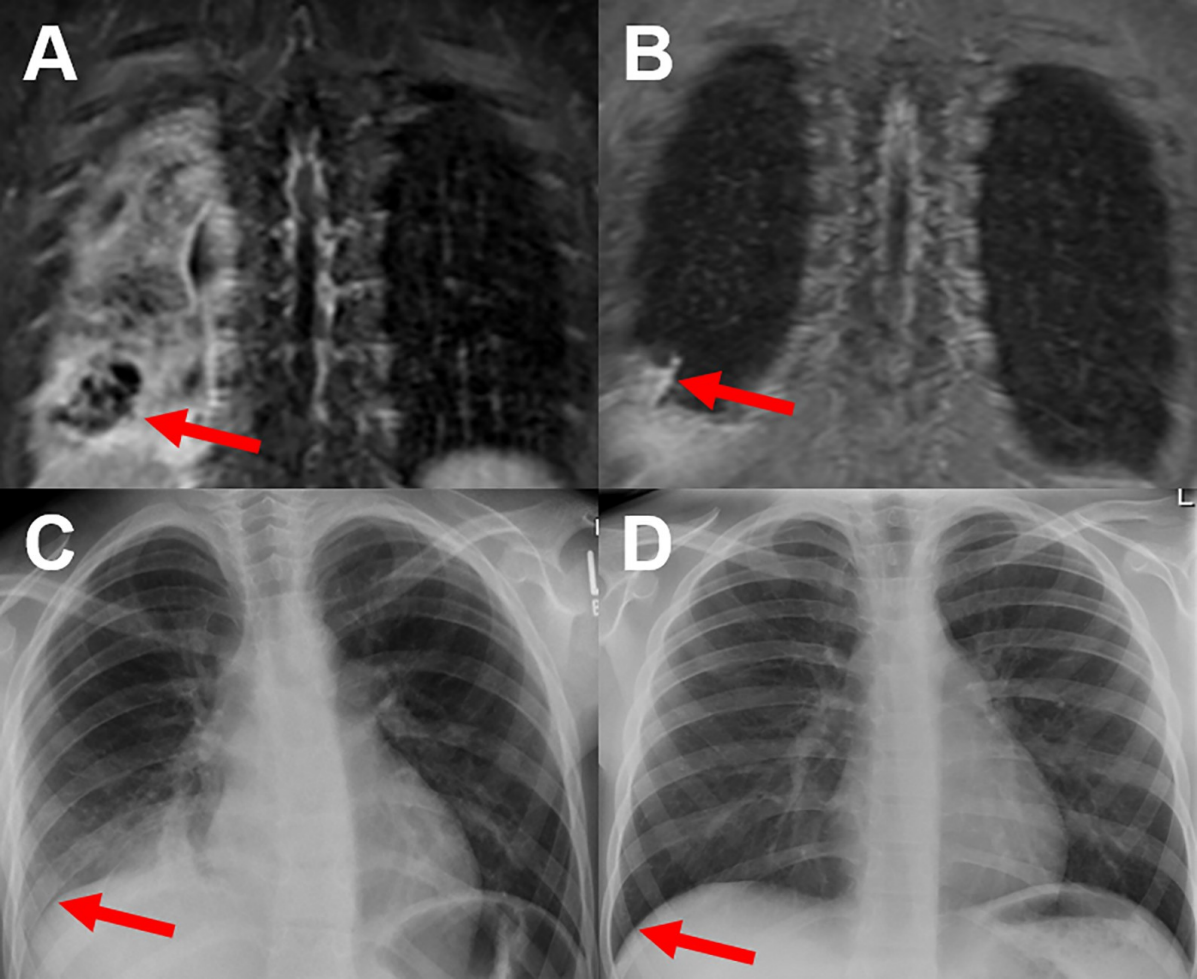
Comparison of T2-weighted sequences and CXR of a 9-year-old child at baseline and follow-up. MRI shows an abscess formation in the right lower lobe at baseline (red arrow) (A), which has regressed under therapy at follow-up (red arrow) (B). The corresponding CXR (C) shows a slight reduction in transparency in the right lower lung field without any clear evidence of an abscess (red arrow). At follow-up, CXR (D) shows no pathological findings not delineating the full extent of residual inflammatory changes (red arrow).
